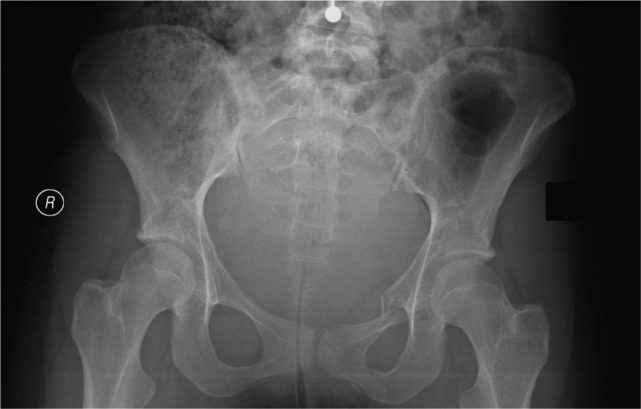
X-ray of pelvis demonstrating fractures of the left superior and inferior pubic rami.
